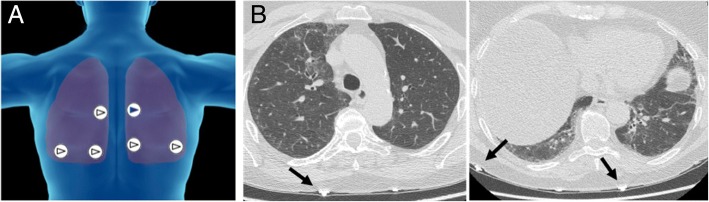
Recording sites selected in the study. Per each side of the chest, two recordings were performed at the lung bases at seven cm below the scapular angle, at both two and five cm from the paravertebral line respectively; another recording was taken from mid chest in correspondence of the fourth or fifth intercostal space, at two cm from the paravertebral line (a). Metallic marks were applied to the posterior chest of the patient and were visible at HRCT (black arrows, (b)
